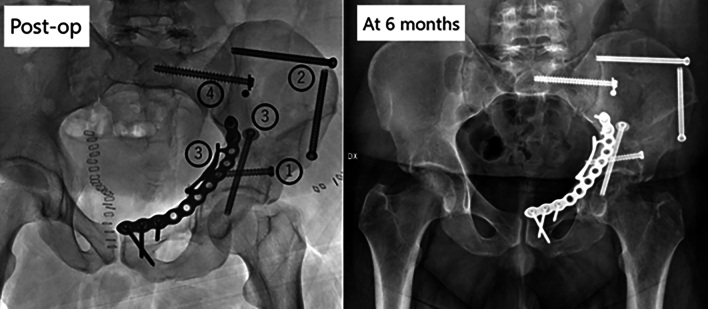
Immediate postoperative X-ray and follow-up at 6 months
